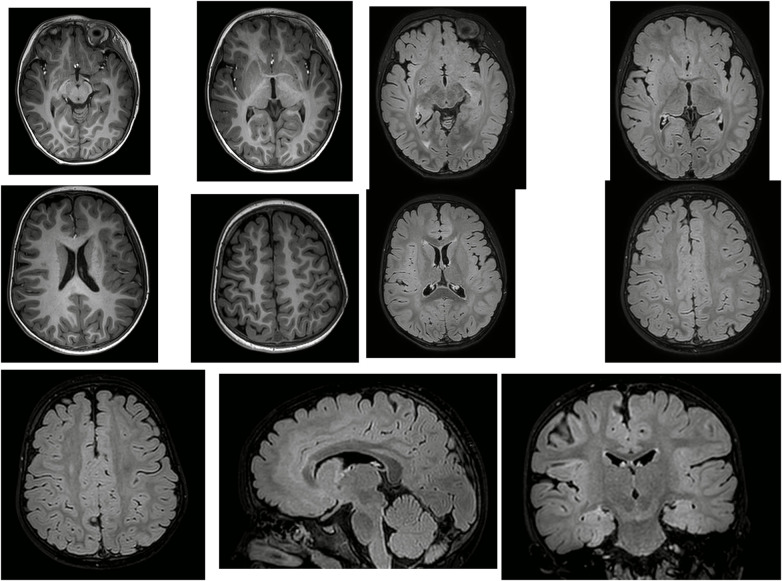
Cranial MRI T1-weighted imaging (T1WI): blurred gray-white matter junction in the left frontal lobe. Cranial MRI (T2FLAIR): irregular hyperintense signals in the left frontal lobe (predominantly medial aspect). Cranial MRI T2FLAIR (sagittal/axial/coronal): diffuse hyperintense signals in the entire left frontal lobe.
